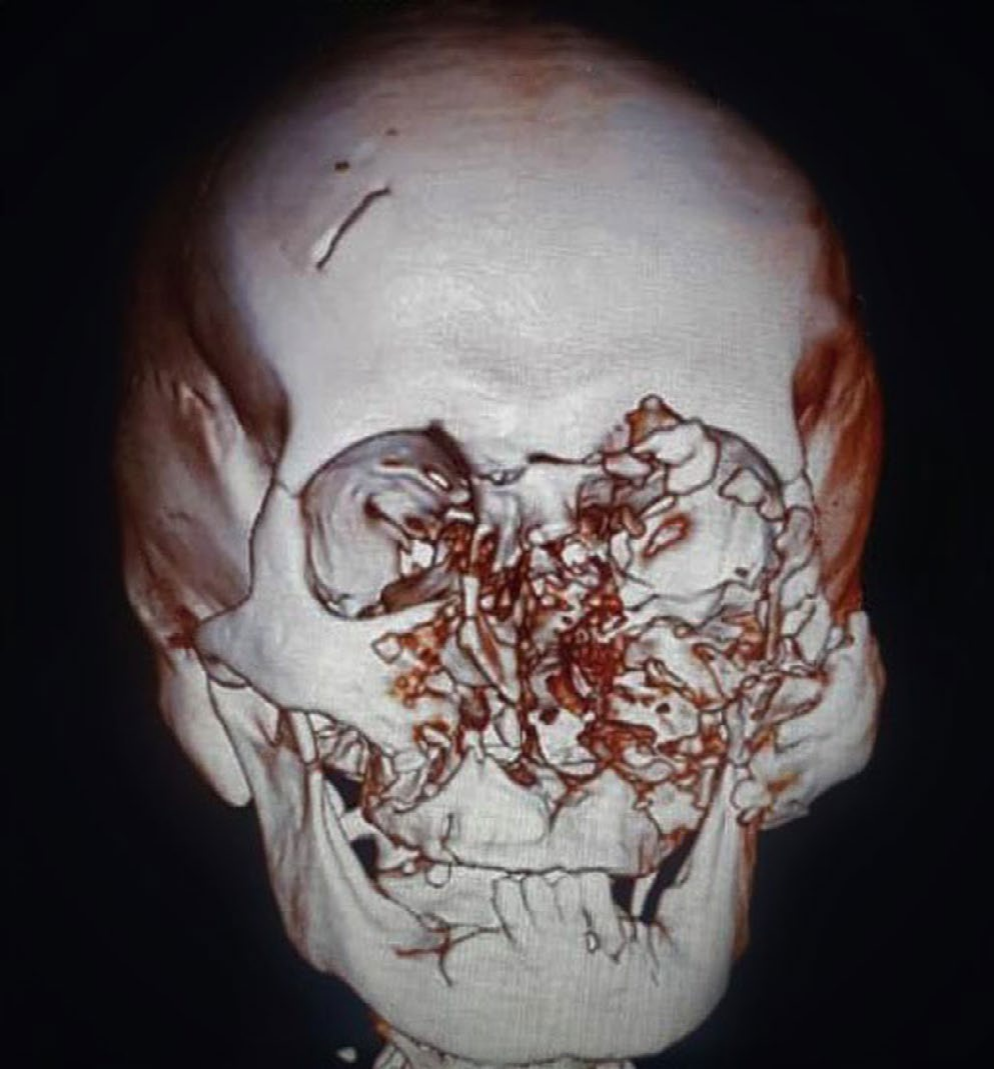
Preoperative three‐dimensional CT scan depicting the extent of fractures
